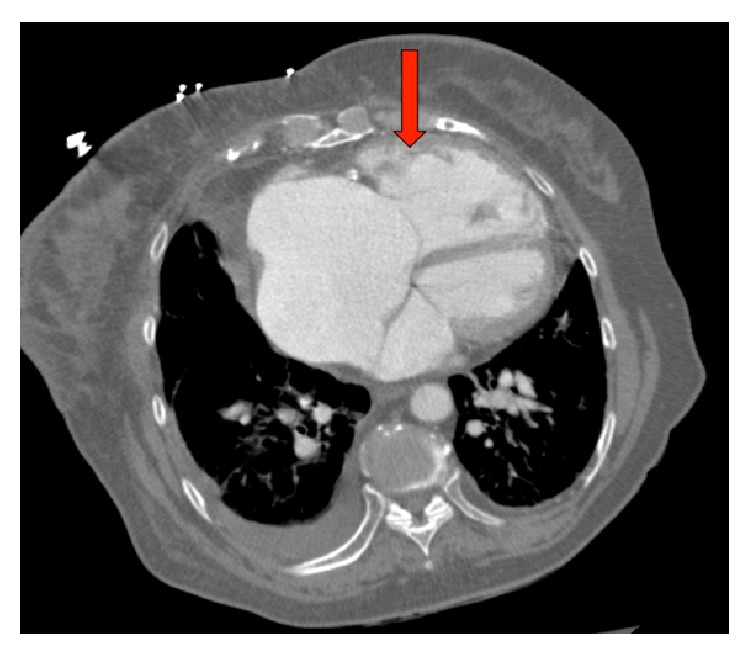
CT-scan showing right ventricular enlargement (red arrow).
